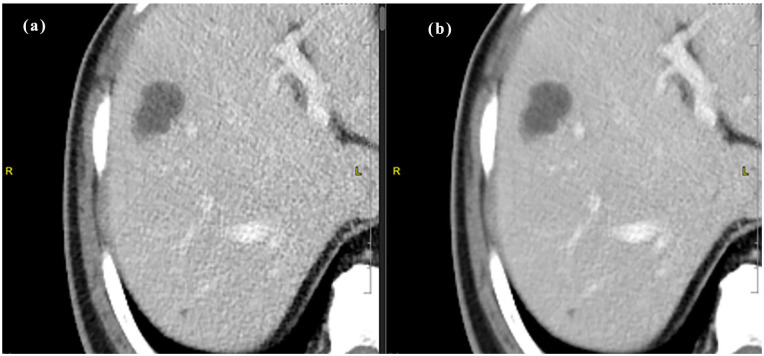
Idose4 (a) versus Precise Image (b) for portal venous phase abdomen and pelvis CT.
